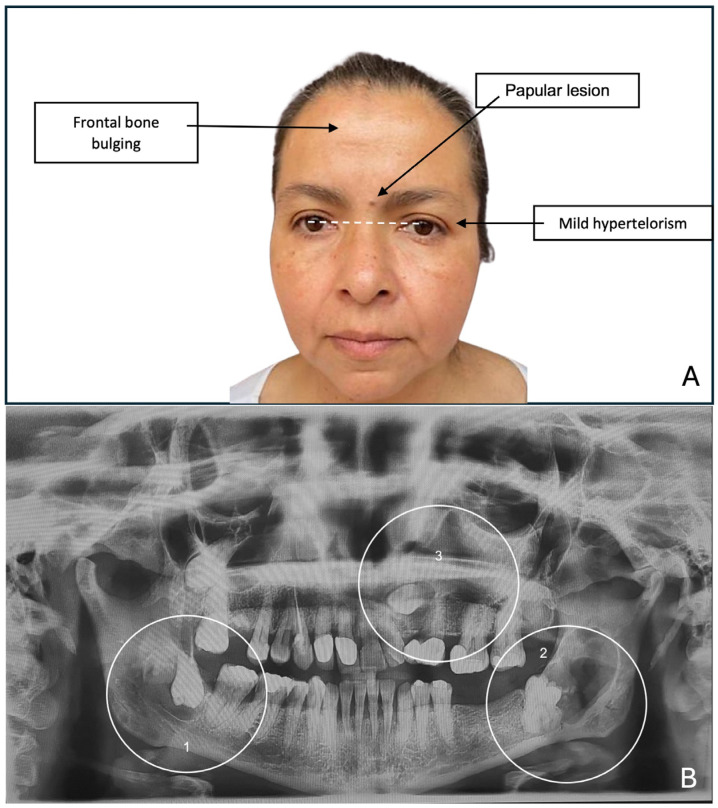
(A) Extraoral photograph of the patient showing thick facies, frontal bone bulging, mild hypertelorism, and a lesion on the skin of the interciliary region. (B) Orthopantomography showing all lesions: 1—mandibular lesion on the right side; 2—mandibular lesion on the left side; 3—lesion involving the maxillary sinus on the left side.
